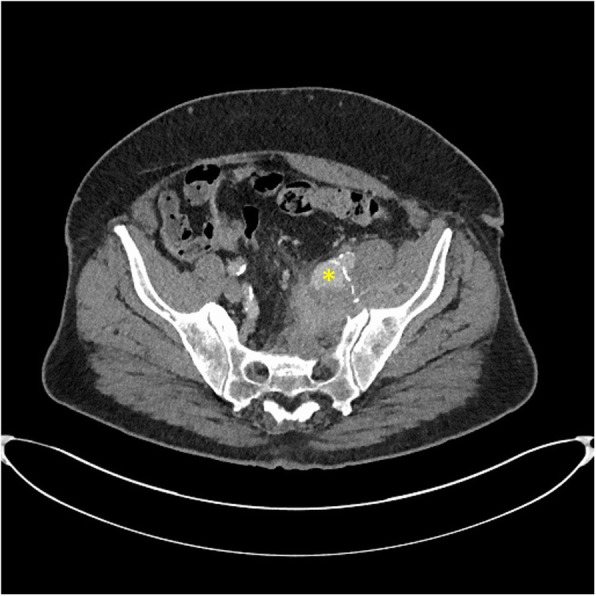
The patient’s contrast CT scan (pelvis) showing a left internal iliac artery mycotic aneurysm (*)
